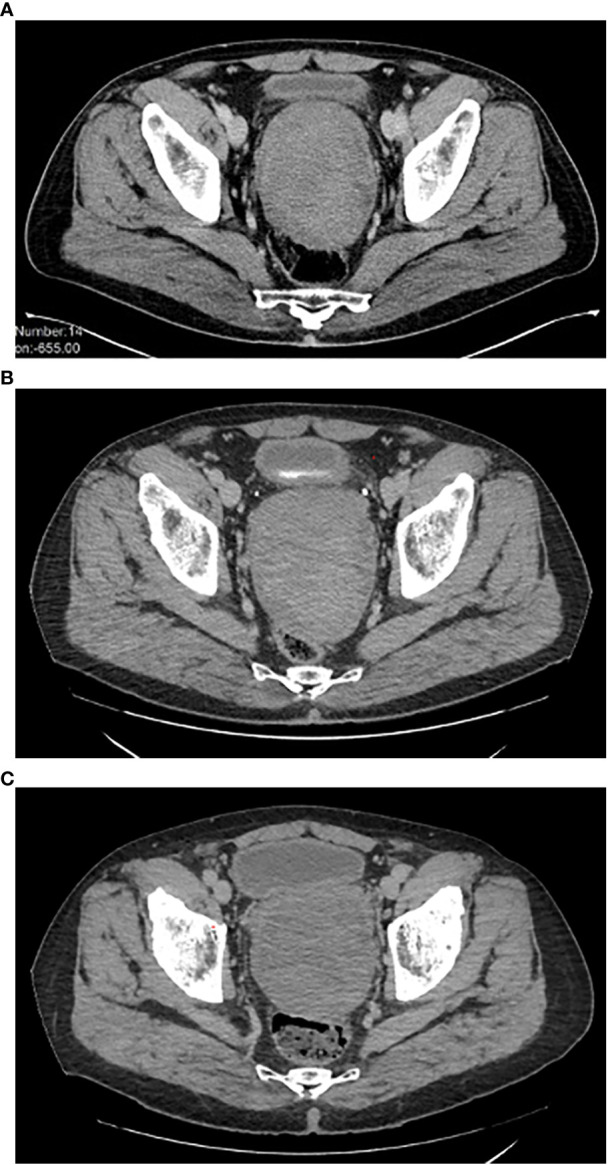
(A-C) Disease imaging (CT-scan). (A) Disease status at diagnosis. (B) Disease status at the time of bicalutamide start. (C) Disease status after 32 months of bicalutamide therapy. CT, computed tomography.
